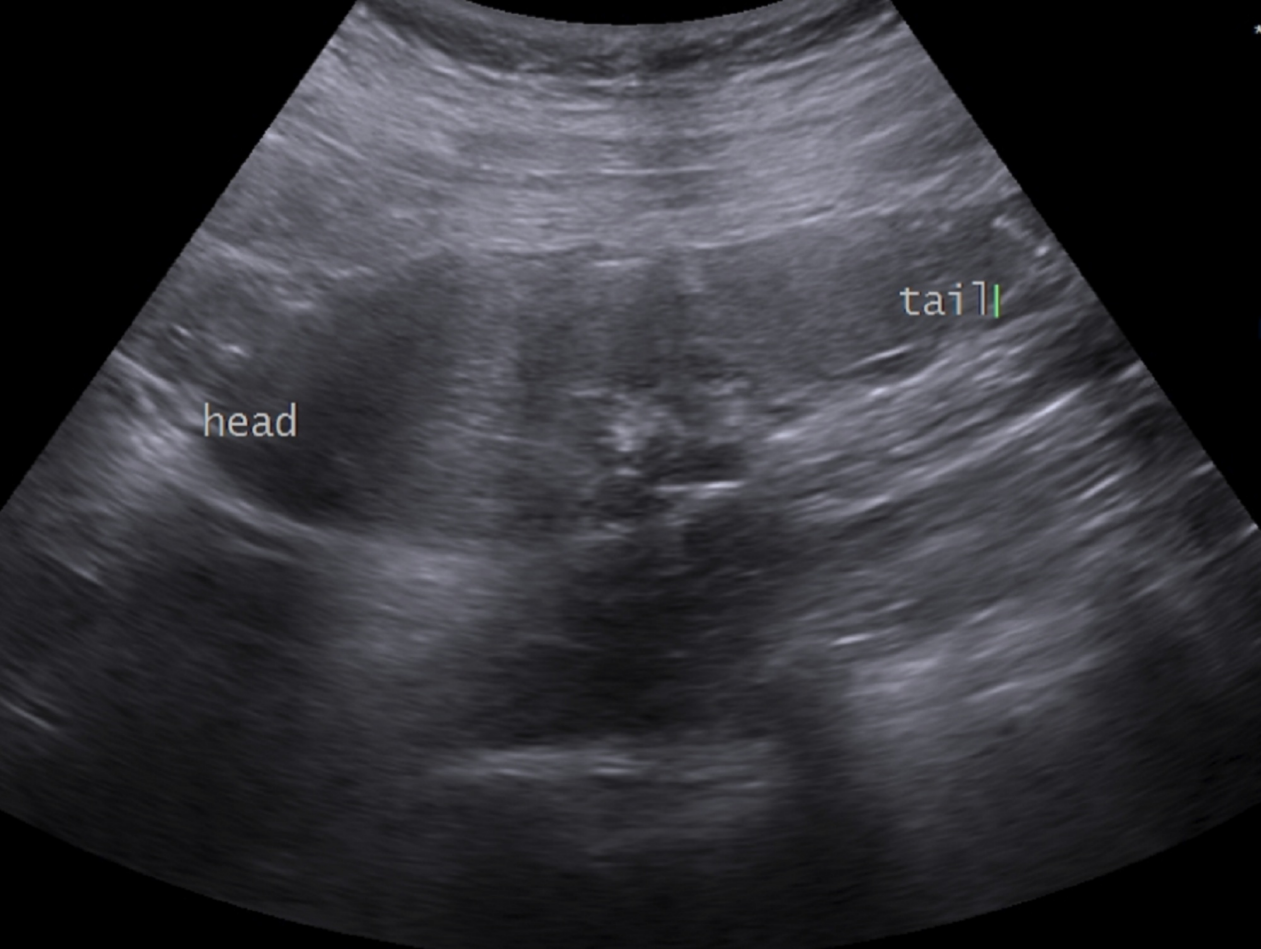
Sonographic craniocaudal measurement of a pancreatic allograft
